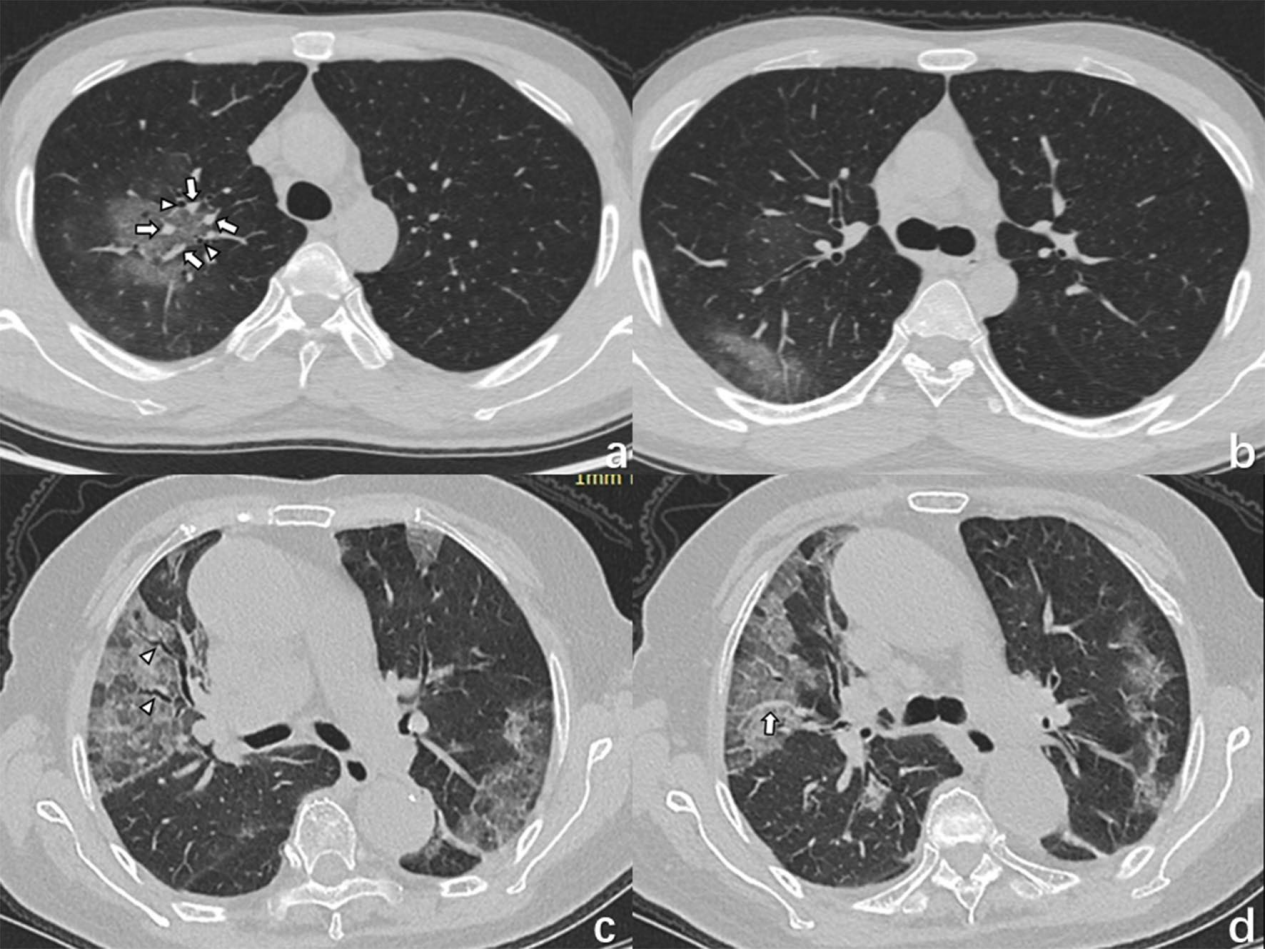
(a, b) A 33-year-old woman with COVID-19 infection, living in Wuhan, presented with fever, rhinocleisis, and dry cough. Axial (a) and coronal (b) CT images showing a subpleural mixed ground-glass opacity with multiple bronchiolectasis (triangles) and small vascular enlargement (arrow) in the right lower lobe. (c, d) A 47-year-old man with a COVID-19 infection, who traveled to Wuhan presented with fever. Axial (c) and coronal (d) CT images shows dominantly posterior- and inferior, multiple, GGOs with bronchiolectasis (triangles) and vascular enlargement (arrows).
